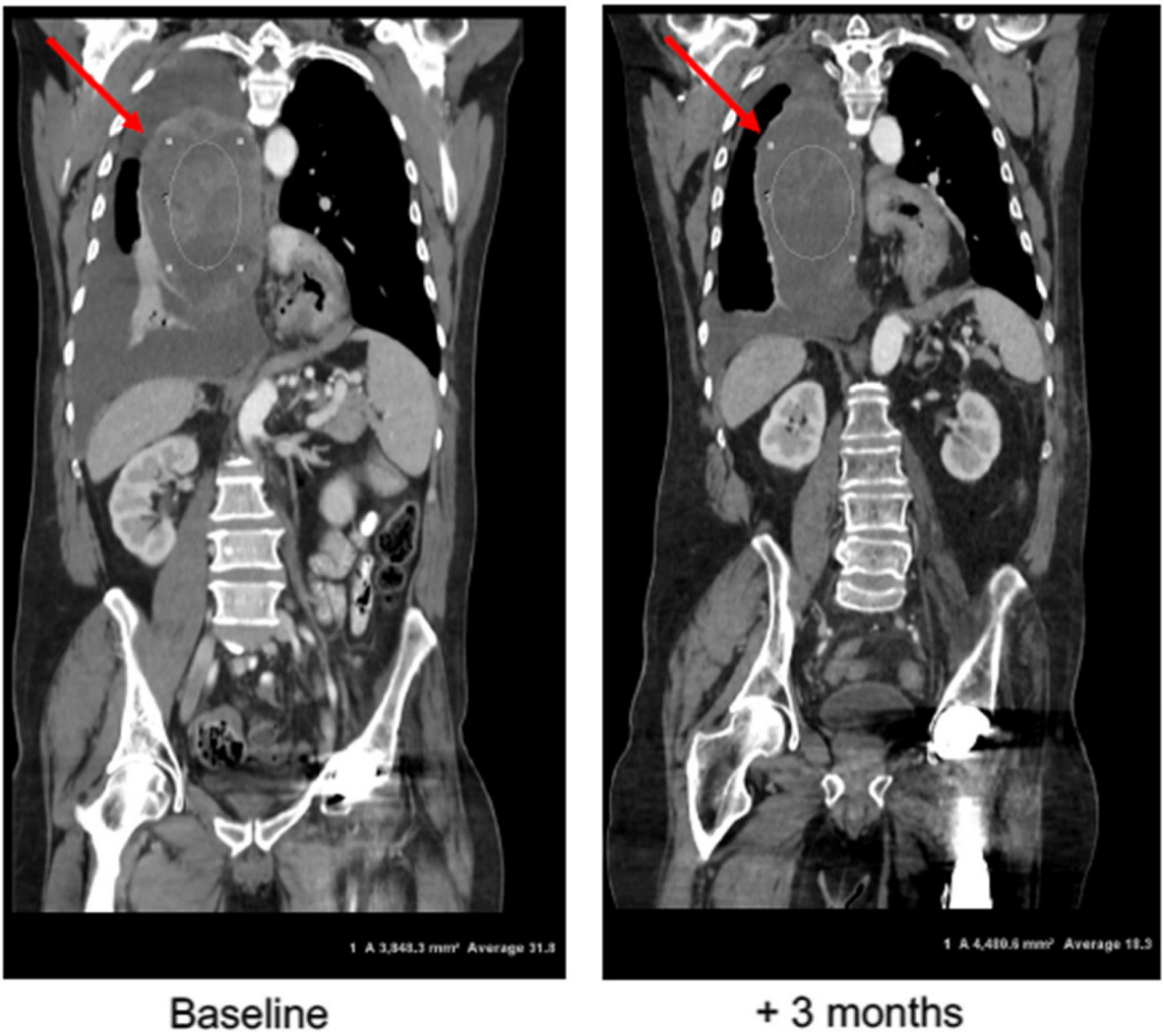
Response to P + S combination therapy in grade II paratracheal chondrosarcoma. Computed tomography (CT) scan. Three months after starting P + S combination therapy a response was observed in the form of tumor liquidification and stabilization of disease
